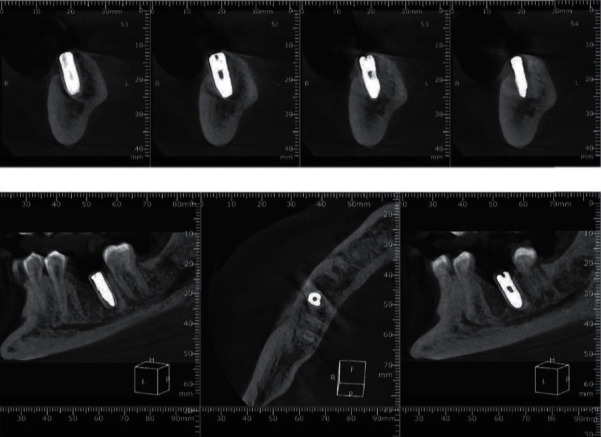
CBCT scanning postoperatively.
